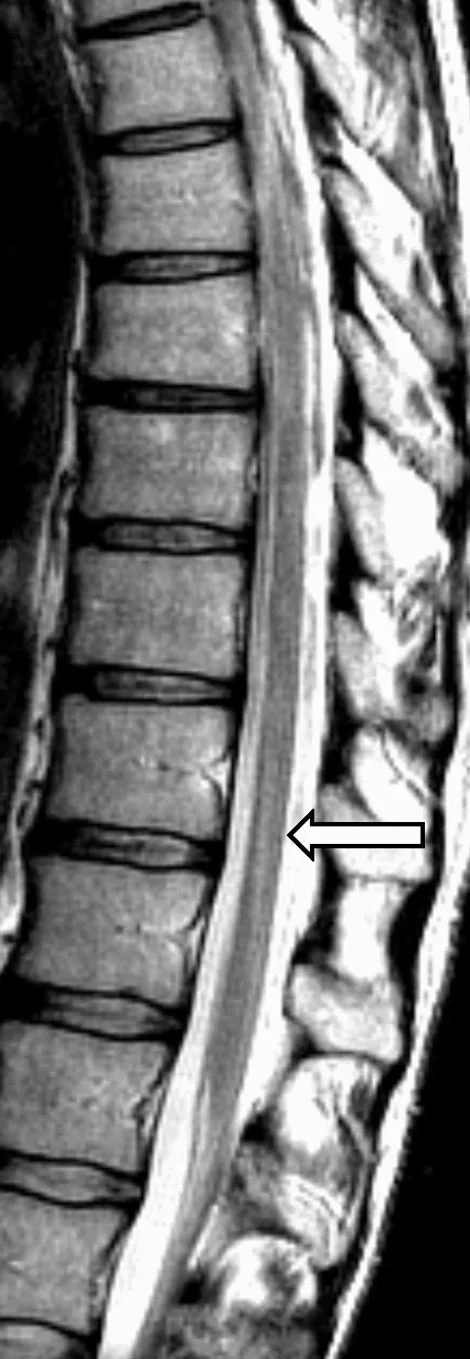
Neuroimaging case #12. Spinal MRI scan 9.5 years after LI. Sagittal T2 weighted sequence showing hyperintense lesion in the lumbar cord (arrow)
